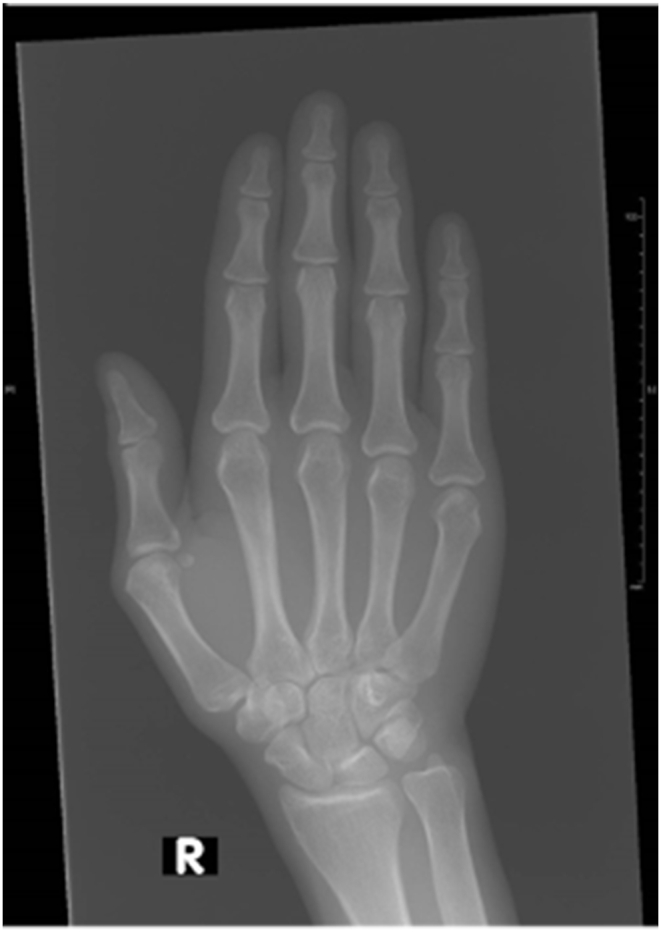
X-ray of the right hand showing only soft tissue swelling in the absence of articular or bony manifestations.
